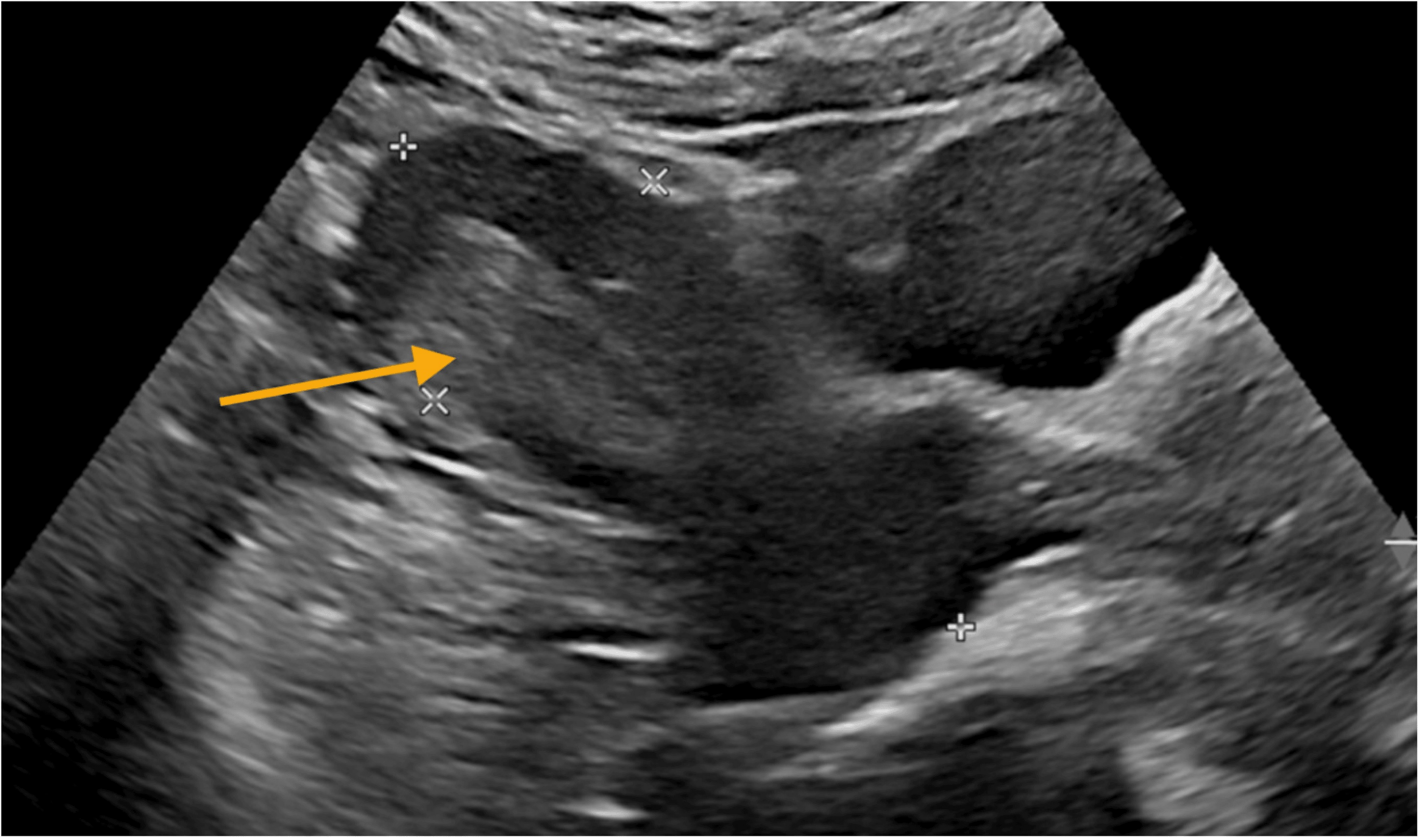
Ultrasound appearance of retained products of conception The associated serum βhCG was 243 mIU/mL.
